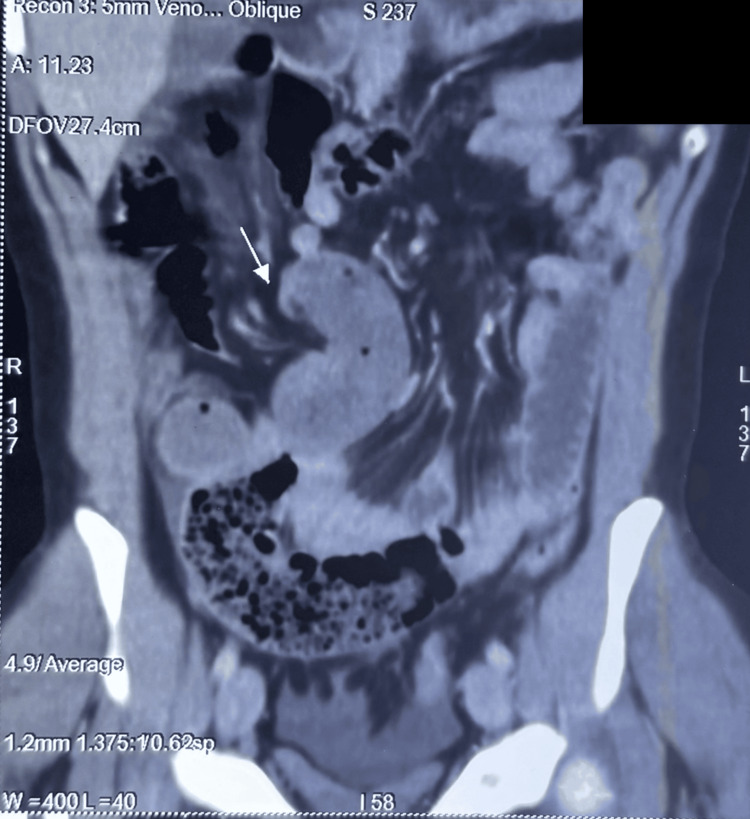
Coronal section of contrast-enhanced computed tomography (CECT) abdomen and pelvis showing dilated small bowel segment (white arrow) indicating closed-loop obstruction
